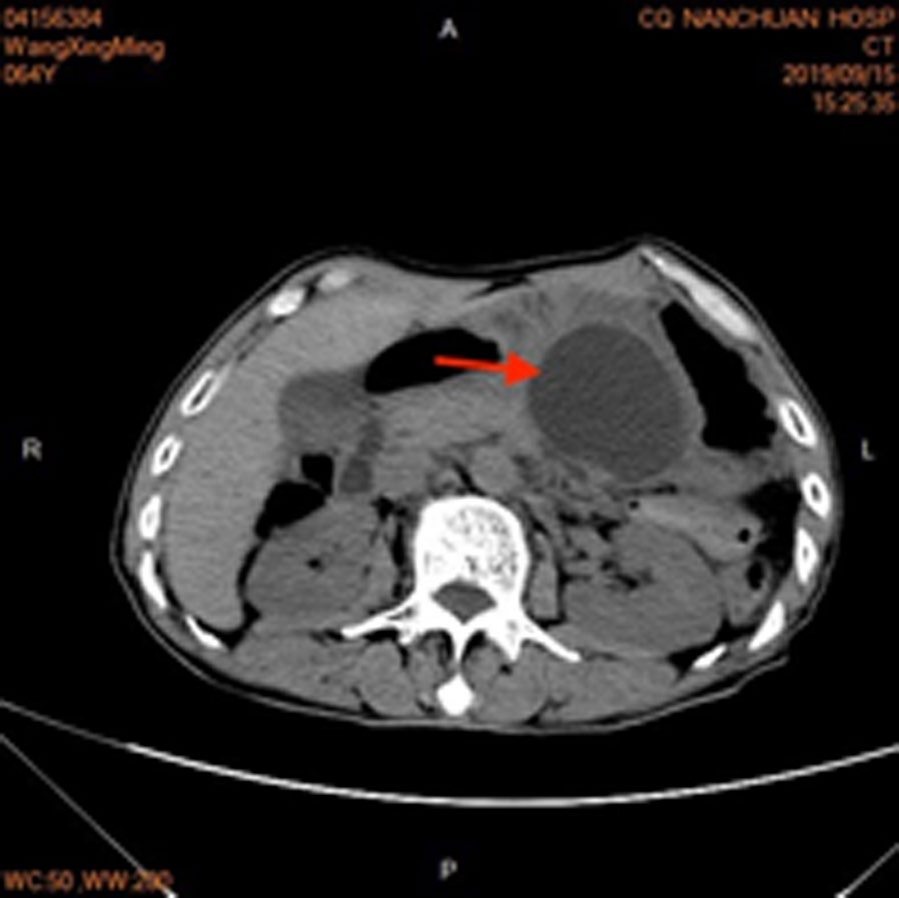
Abdominal CT revealed pancreatitis with a pseudocyst (red arrow) with a diameter measuring 7 cm
